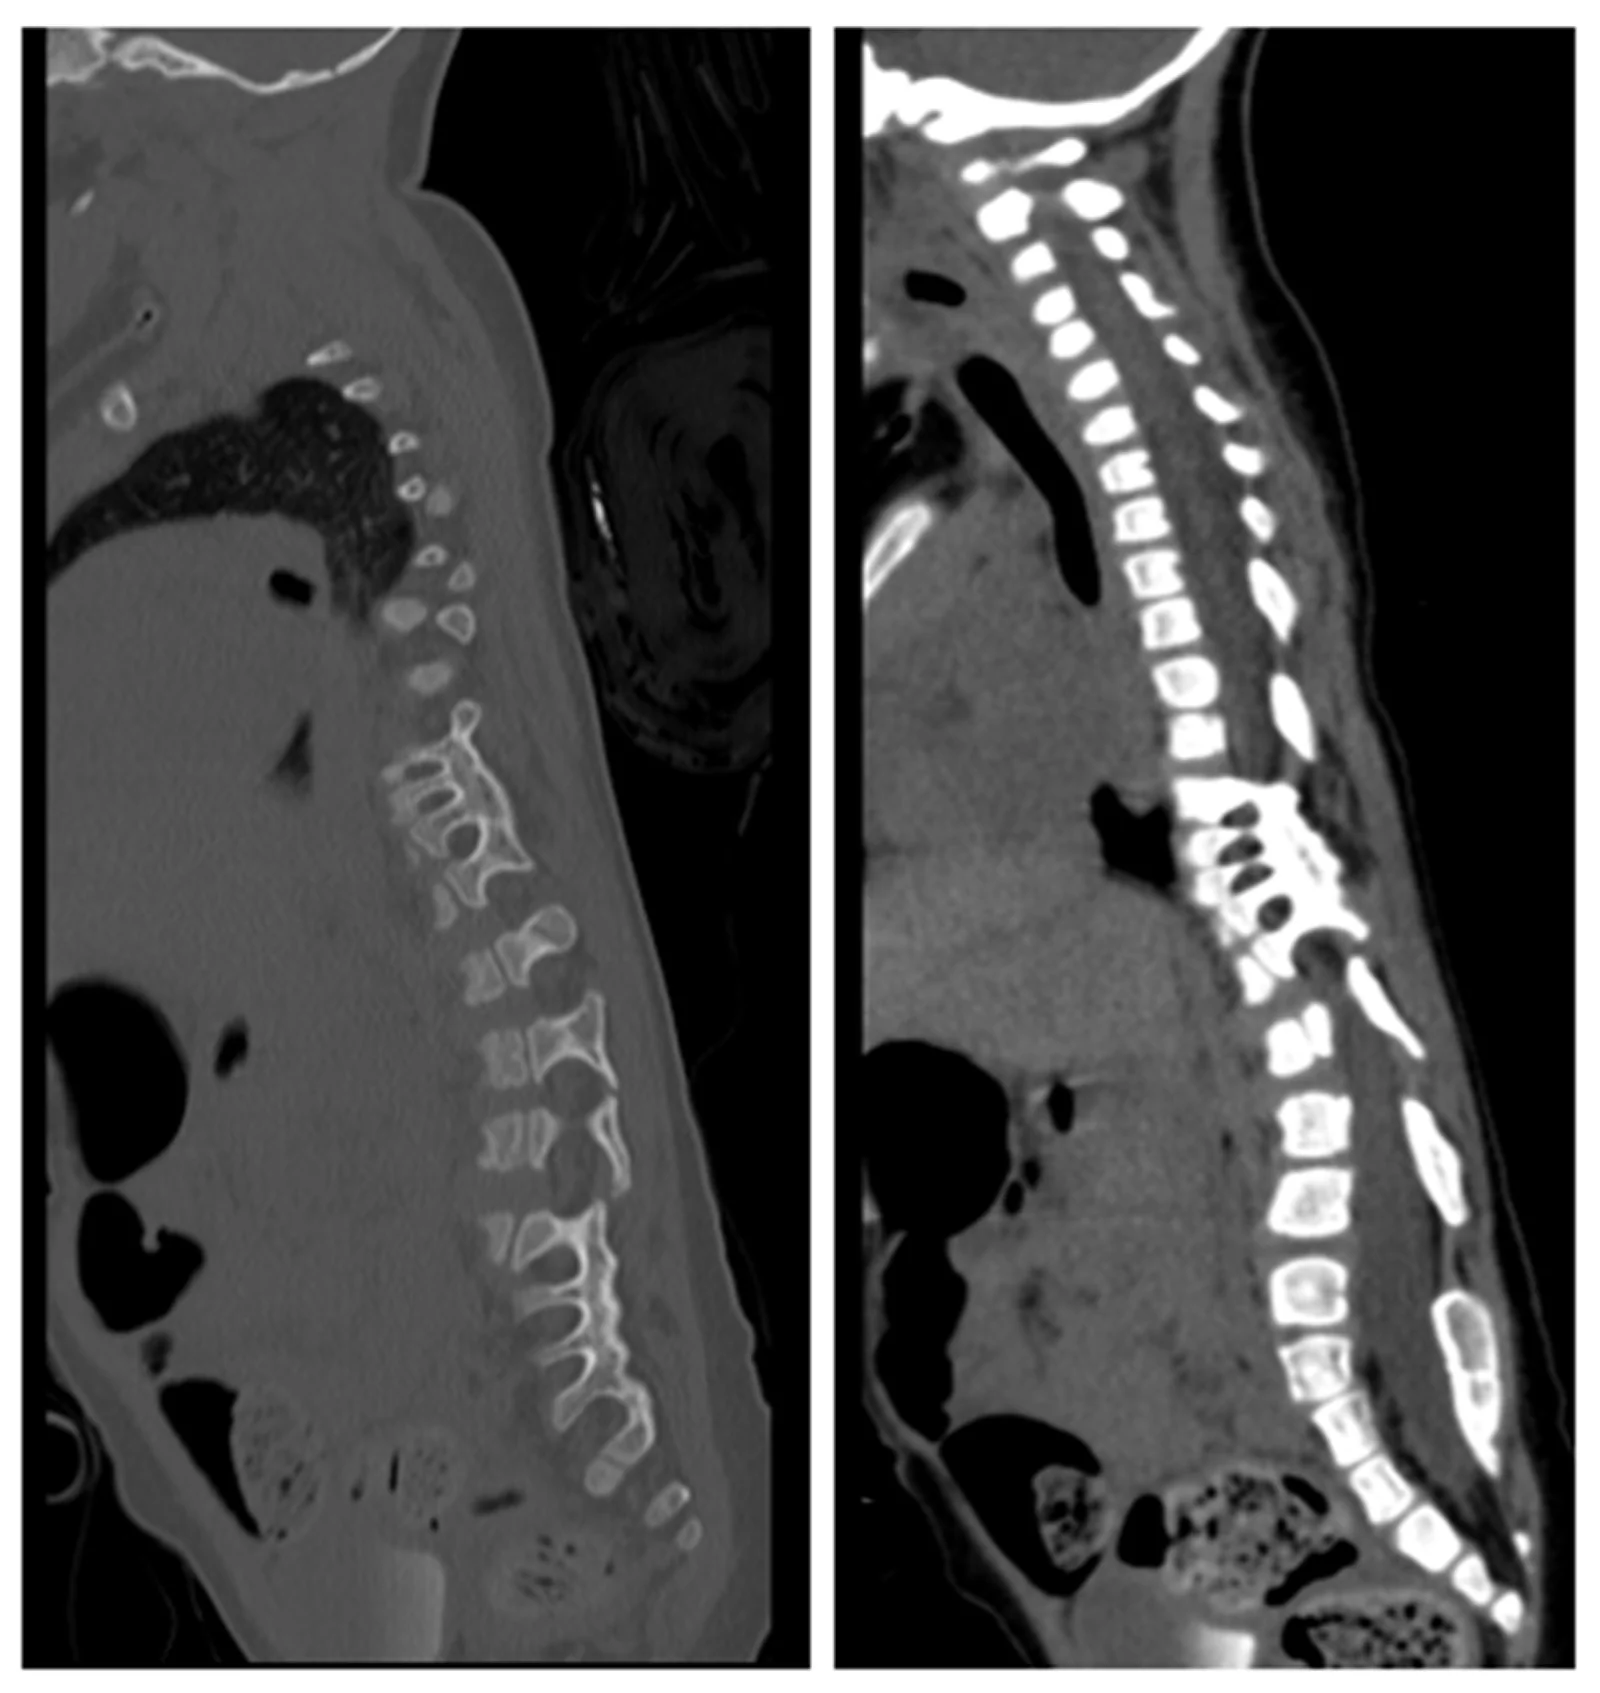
Spinal MRI (left) and CT scan (right) of the index patient at 18 months of age. MRI demonstrates multisegmental fusion of posterior vertebral elements from D1 to D11 and L1 to S2, with posterior arch clefts at C1–C2, D1–D2, D5–D11 and the sacral bodies, as well as coccygeal dysgenesis. CT confirms the complex pattern of vertebral segmentation anomalies and allows detailed assessment of posterior element involvement. A left-convex scoliotic curve is present at the thoracolumbar junction with kyphotic deformity from D10 to L2.
